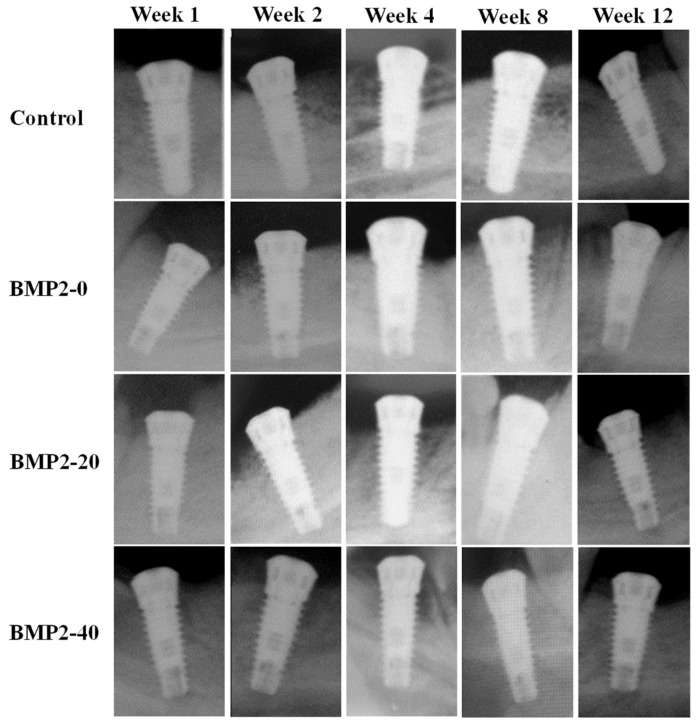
Radiographic images from the investigated implants after implantation for different durations.
